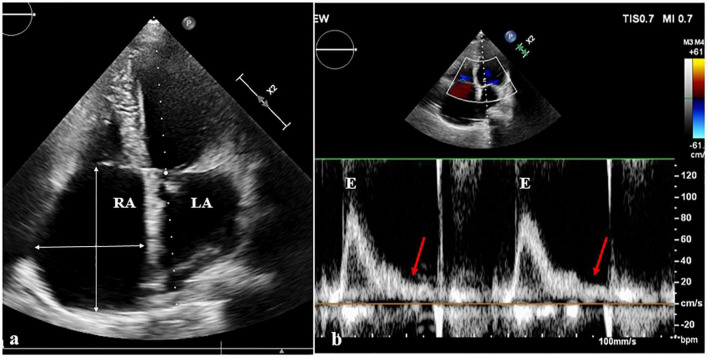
Echocardiography images. (a) On the echocardiography, both atrium were observably enlarged, the right atrium (RA) size was 8.9 cm × 5.6 cm, and the left atrium (LA) size was 7.2 cm × 5.6 cm. (b) In the mitral inflow pulse by Doppler recording, only E wave was observed in the early diastolic period, but no A wave was observed in the late diastolic period.
